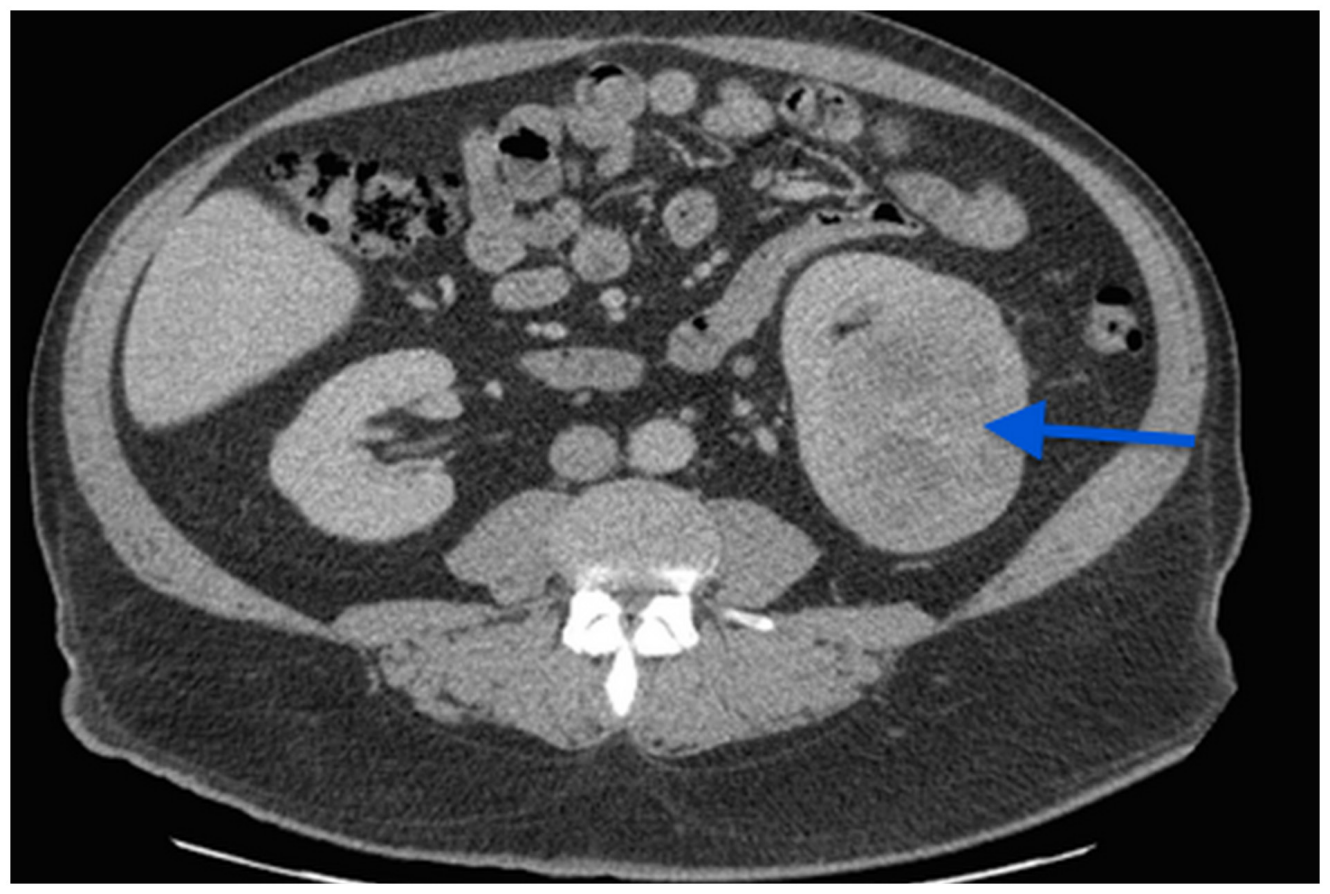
A CT scan image showing a 7 cm left kidney mass.
